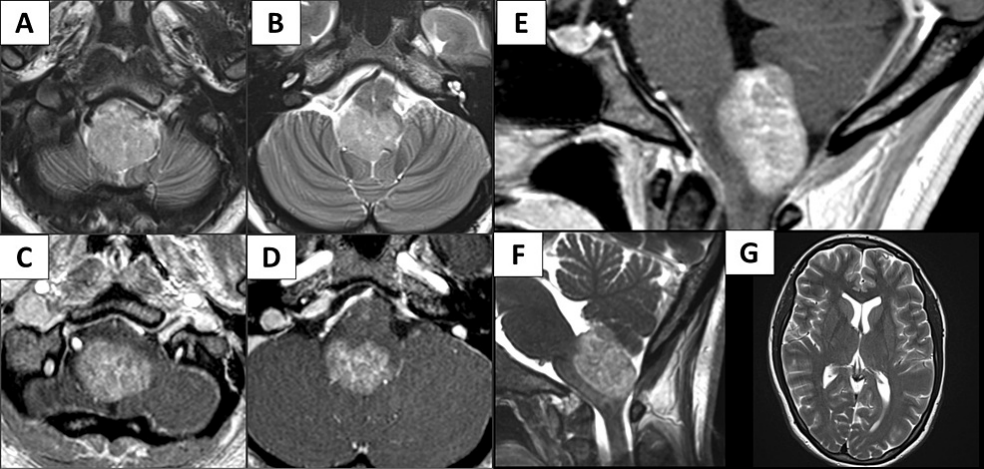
Pre-operative images of the representative case Magnetic resonance imaging revealed a solid mass occupying the medulla oblongata (A). The lesion exhibited T2 high intensity and was compressing the surrounding structures (B). Homogenous gadolinium enhancement was observed (C, D). The lesion was located at the dorsal aspect of the medulla oblongata and was protruding to the fourth ventricle (E, F). Hydrocephalus was not evident (G).
